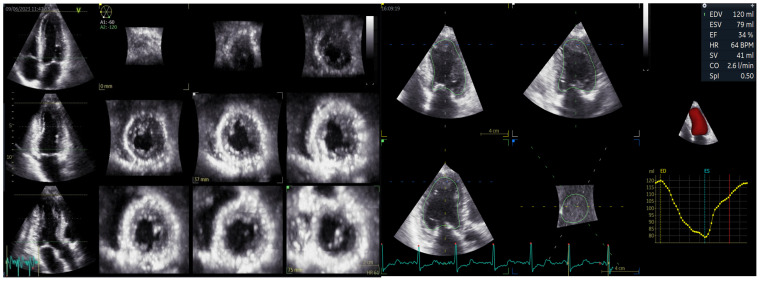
Measurements of left ventricular volumes and ejection fraction with three-dimensional echocardiography using the 4D auto LVQ software Vivid GE E95 System.
